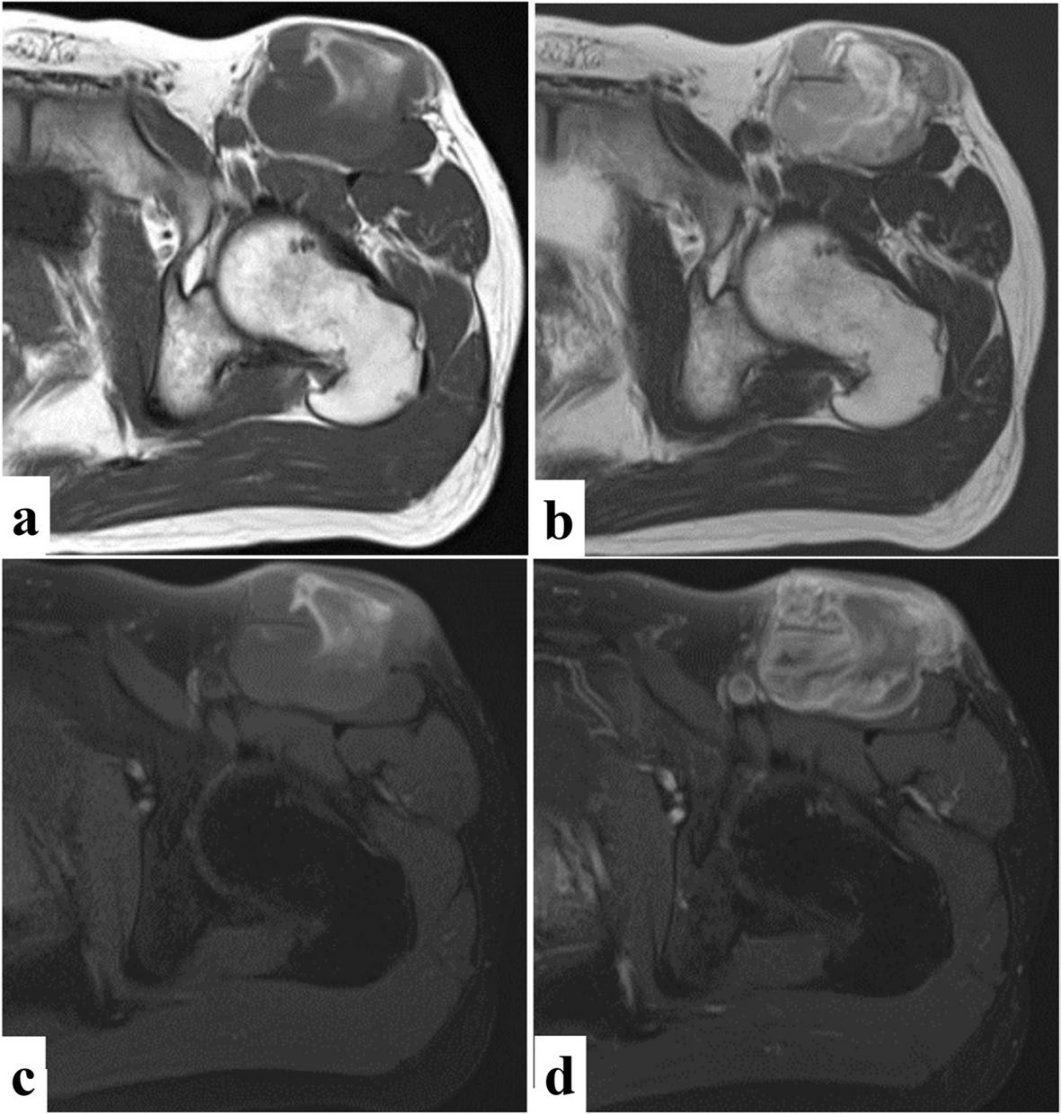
Magnetic resonance images. Magnetic resonance imaging shows a mass lesion in the left femur. The tumor occupying the sartorius muscle has a Hypointensity with few hyperintensities on T1-weighted image (WI) and b Hyperintensity on T2WI. c Fat-saturated T1WI shows some areas of hyperintensity in the mass, and d Gadolinium-enhanced fat-saturated T1WI shows enhancement in the mass lesion excluding the central region
